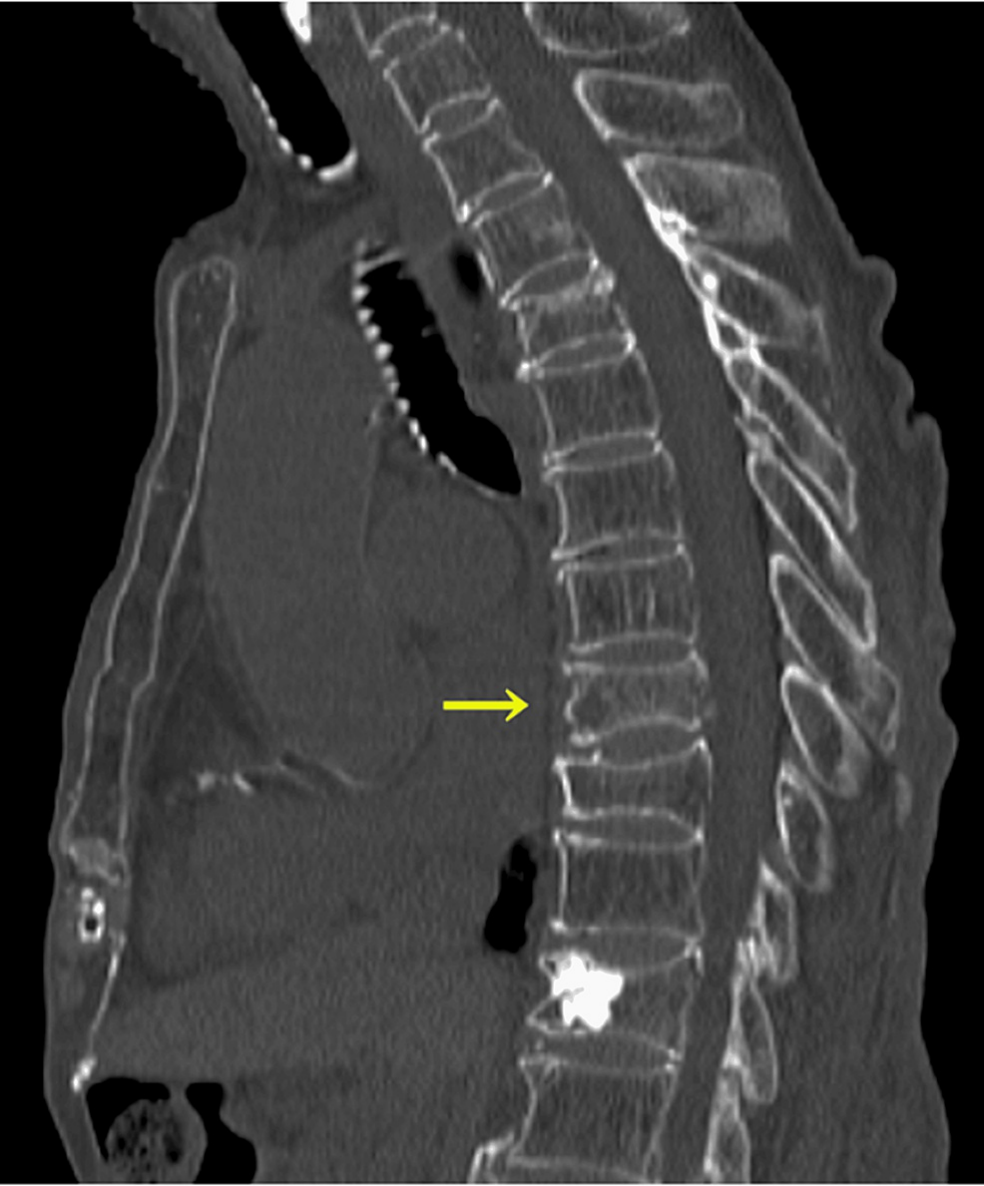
Computed tomography showing a thoracic spine fracture (yellow arrow).
